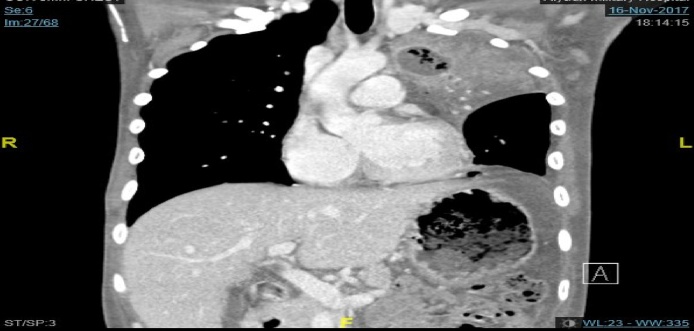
CT chest coronal view showing left upper lobe consolidation and cavitation.
